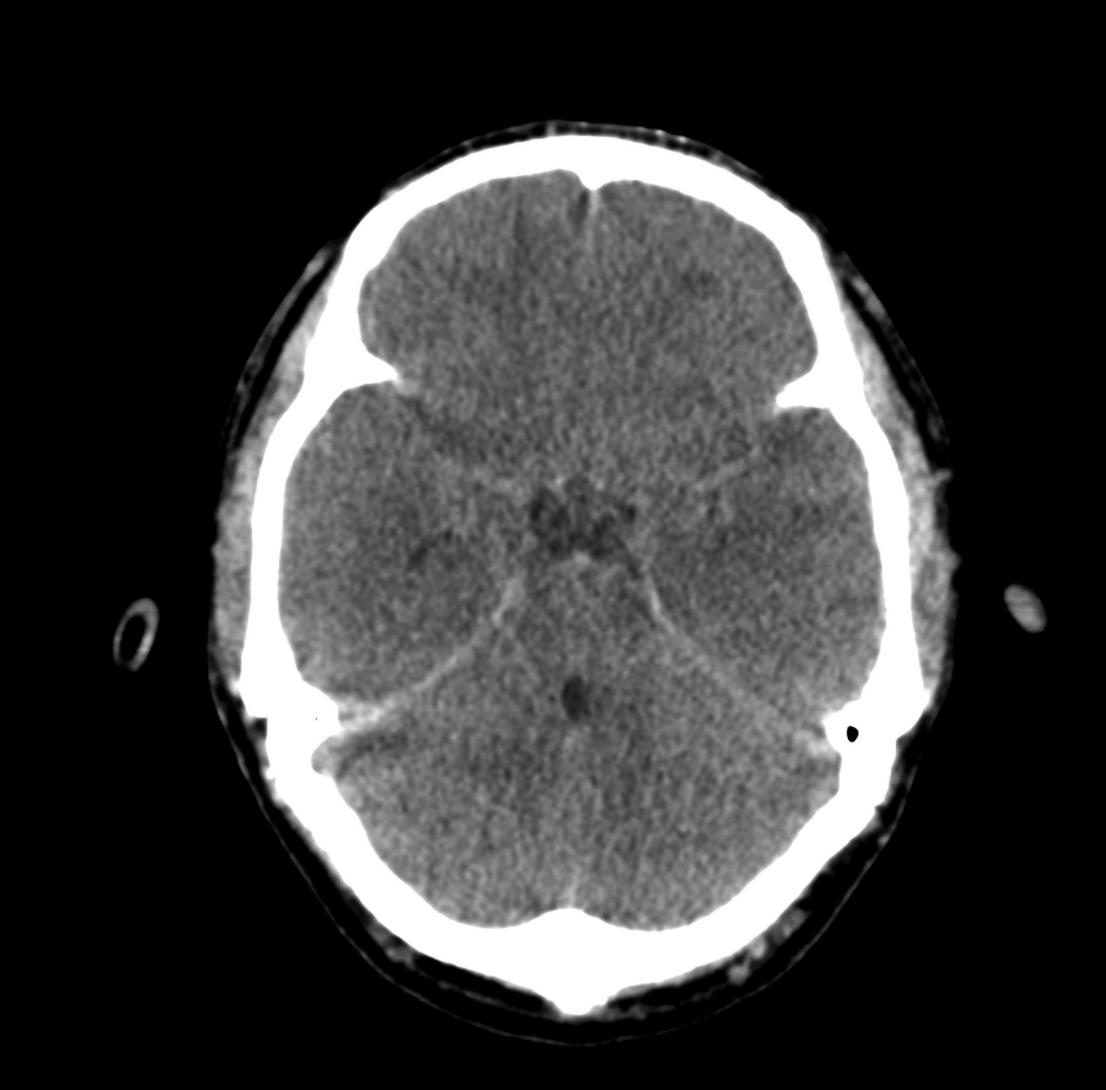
CT scan done 24 h later showing markedly increased cerebral edema with evidence of bilateral uncal herniation.
